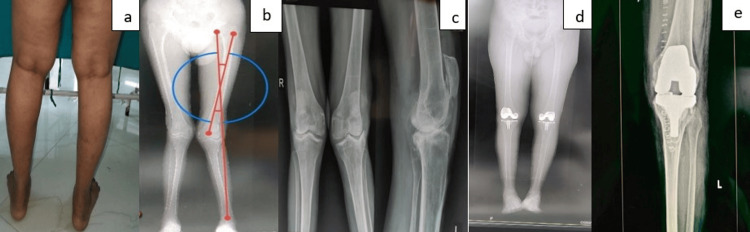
Case 5 (a) Preoperative clinical photograph. (b) Preoperative radiograph. (c) Preoperative scanogram. (d) Postoperative scanogram. (e) Postoperative radiograph.
